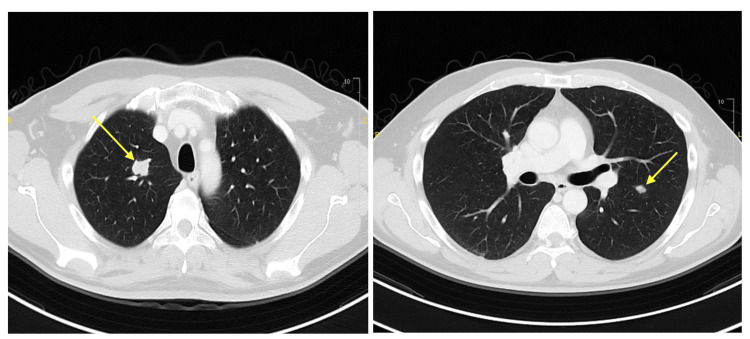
Axial view of CT thorax showing bilateral pulmonary nodules suggestive of metastatic disease (arrows) CT: computed tomography
